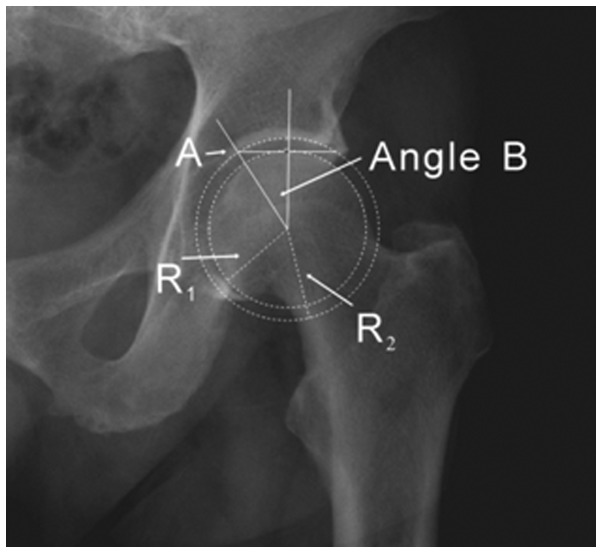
Radiograph showing the relative position of point A. Point A is used to measure the modified Tönnis angle, and the relative position of point A is determined by angle B. R_1_ and R_2_ represent the radii of the femoral head and acetabulum, respectively. Theoretically, cosB = R1/R2; therefore, the relative position of point A can only be affected by the radii of the femoral head and acetabulum.
